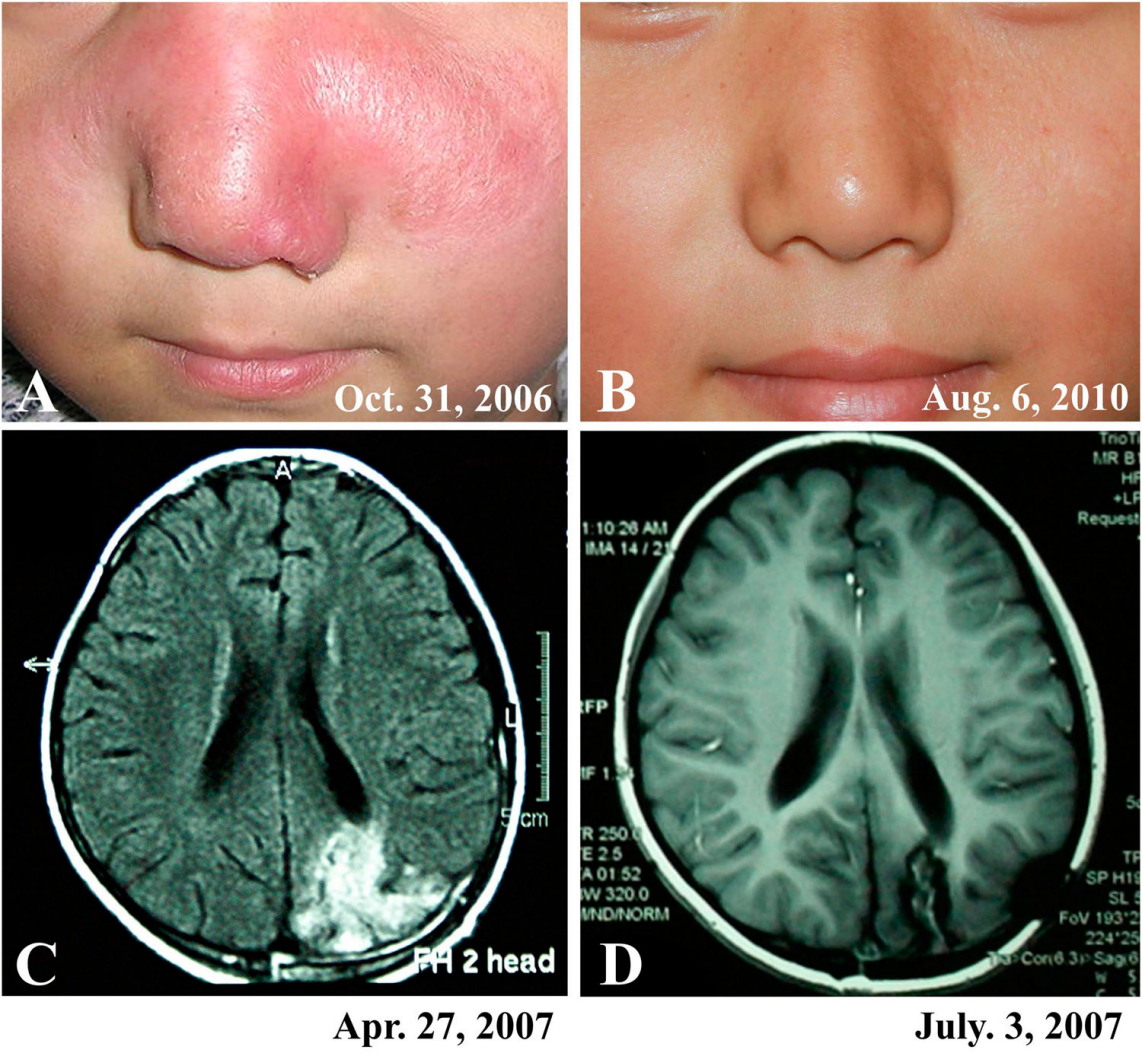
A patient (case 8) with cutaneous and encephalic *Balamuthia mandrillaris* infection survived after treatment with brain surgery and multiple medicines. A: The patient showed red plaque on the face. B: The patient was free of disease at the 4-year follow-up. C: MRI before treatment showed an irregular patchy high signal area in the left occipital lobe. D: Postoperative MRI showed a partial defect of the left occipital bone and partial absence of the left occipital lobe.
